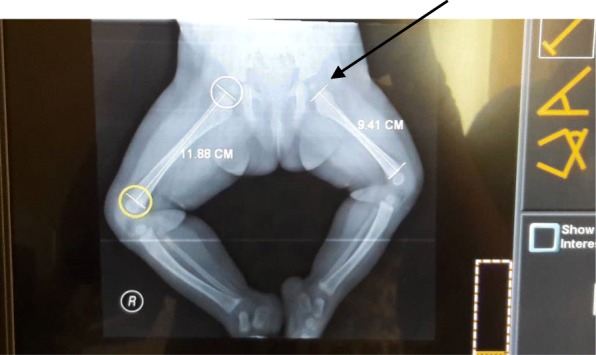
Frog-leg lateral radiograph of the pelvis and lower limbs of Case 2 showing a normal but short left femur with shortening of 2.5 cm. Note that the acetabulum and femoral capital epiphysis were well developed bilaterally (*arrow*)
